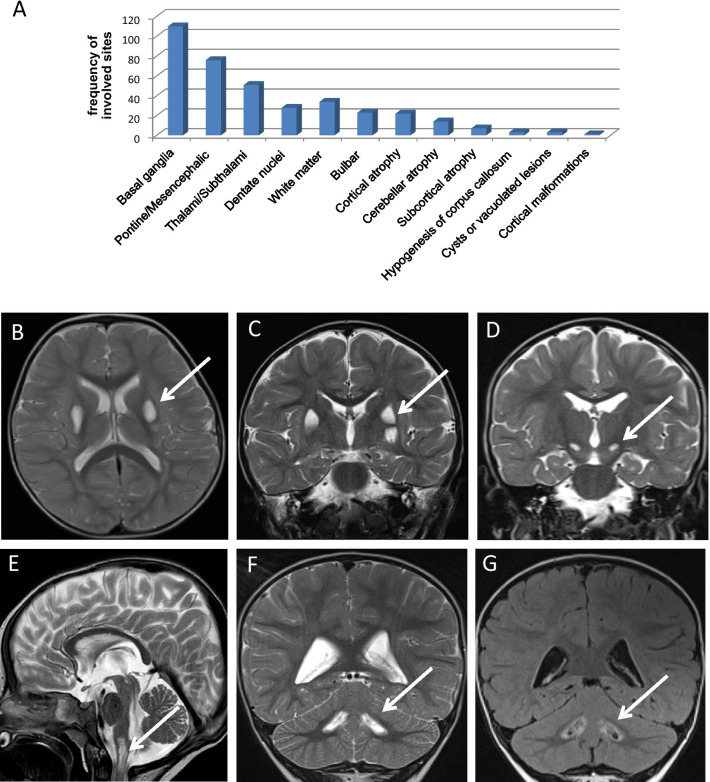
MRI findings. Graph depicts frequency of brain MRI involved regions in our LS cohort (A). Supratentorial pattern: axial (B) and coronal (C) T2WI sequences show hyperintensities of putamen in a patient with complex I deficiency associated to *MT-ND3*; supra and subtentorial pattern: coronal (D) and sagittal (E) T2WI sequences show hyperintensities in subthalami (D) and brainstem (E) in a patient with complex IV deficiency associated to *SURF1*; subtentorial pattern: coronal T2WI (F) and FLAIR (G) show hyperintensities (F) and cavited pattern (G) in dentate nuclei in a patient with complex I deficiency associated to *NDUFAF6*
